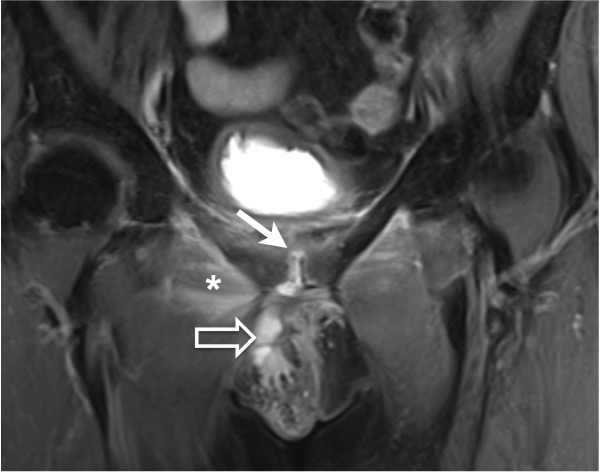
Fat suppressed proton-density-weighted MRI sequence in the coronal plane shows hypersignal intensity on the pubic symphysis suggesting synovitis (arrow). These signal abnormalities seemed to be continuous with a voluminous abcess measuring 48 x 22 x 8 mm extending to the external genitalia (open arrow). Edematous infiltration of the right adductor (asterisk) is also shown.
